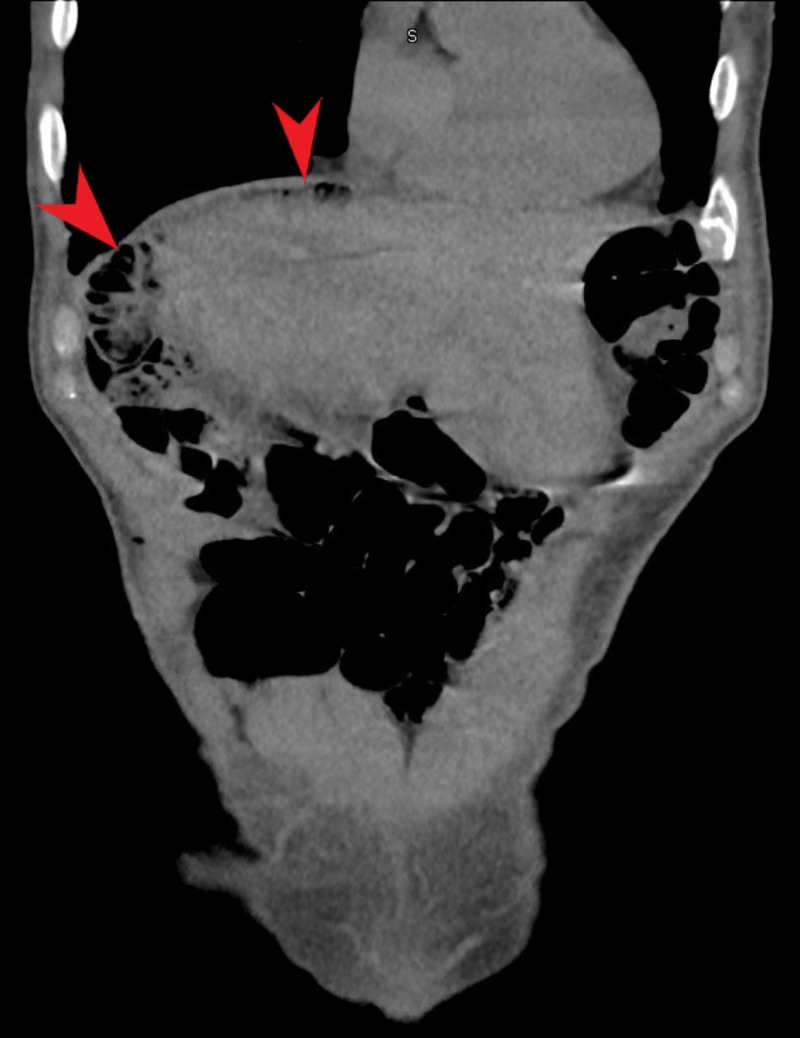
CT of the abdomen. Red arrows show loops of bowel in the right subdiaphragmatic space.
